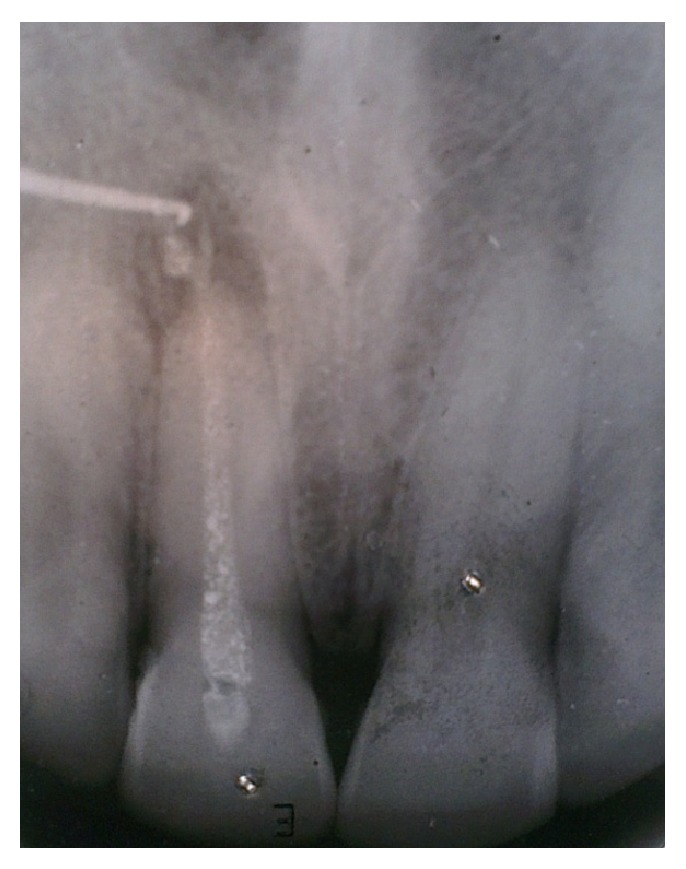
Radiograph showing gutta percha tracing of the sinus tract.
